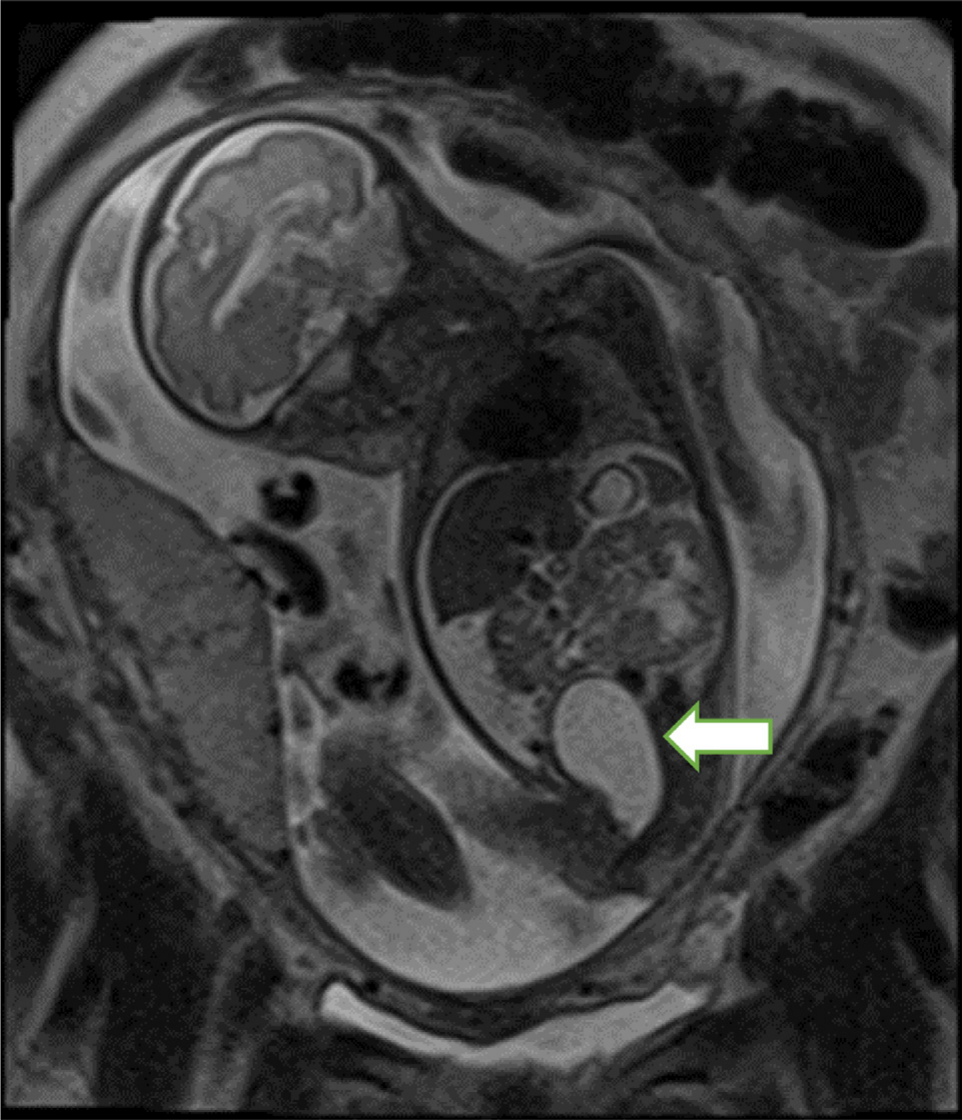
Fetal MRI at 27w6d showing ovoid pelvic cystic structure representing dilated fluid filled vagina (white arrow).
